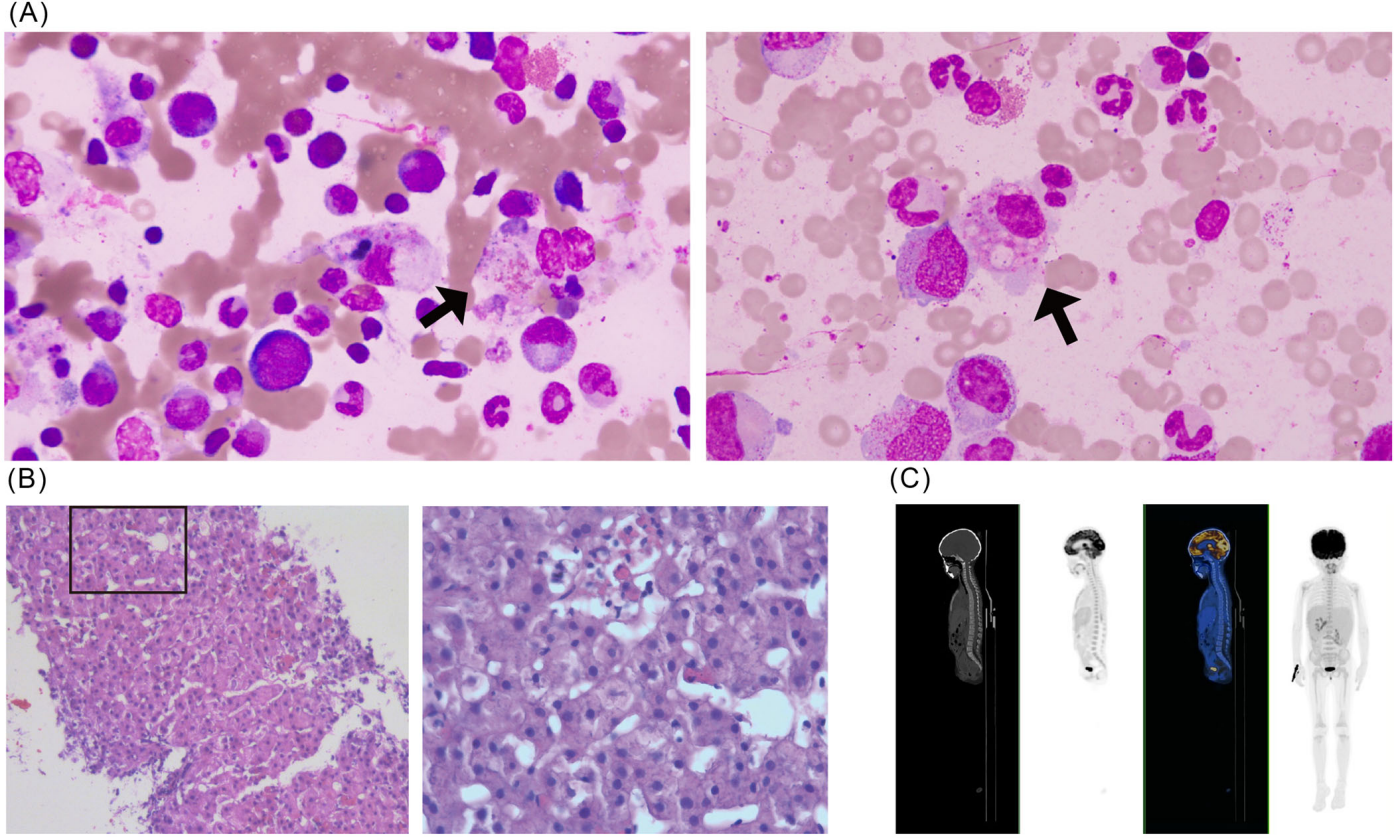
Pathological and radiological evidence supporting the diagnosis of HLH. (A) Bone marrow showed foamy macrophages engulfing blood cells; (B) liver biopsy indicated hepatocytes swelling and ruled out acute allograft rejection; (C) PET‐CT scan showed no evidence of proliferative disorders and lymphoma. HLH, hemophagocytic lymphohistiocytosis; PET‐CT, positron emission tomography‐computed tomography.
